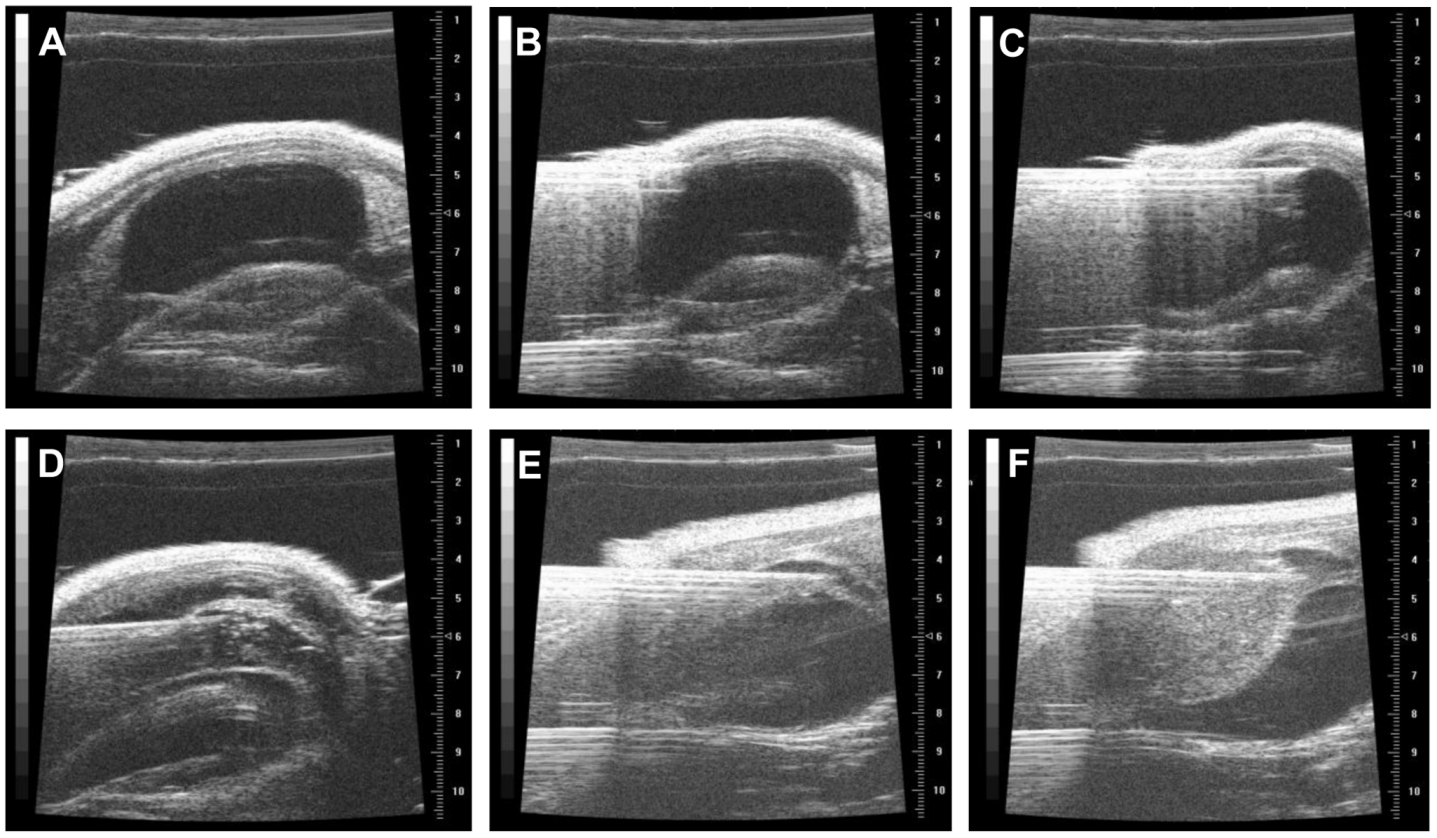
Inoculation of tumor cells. A. Detection of the needle on the ultrasound screen. B. Perforation of the skin and abdominal wall muscles. C. Needle insertion into the bladder wall without penetration of the mucosa. D. Injection of PBS (50 µl) between the muscular layer and the mucosa. E. Guidance of second needle into the artificially created space. F. Injection of tumor cells suspended in Matrigel®.
